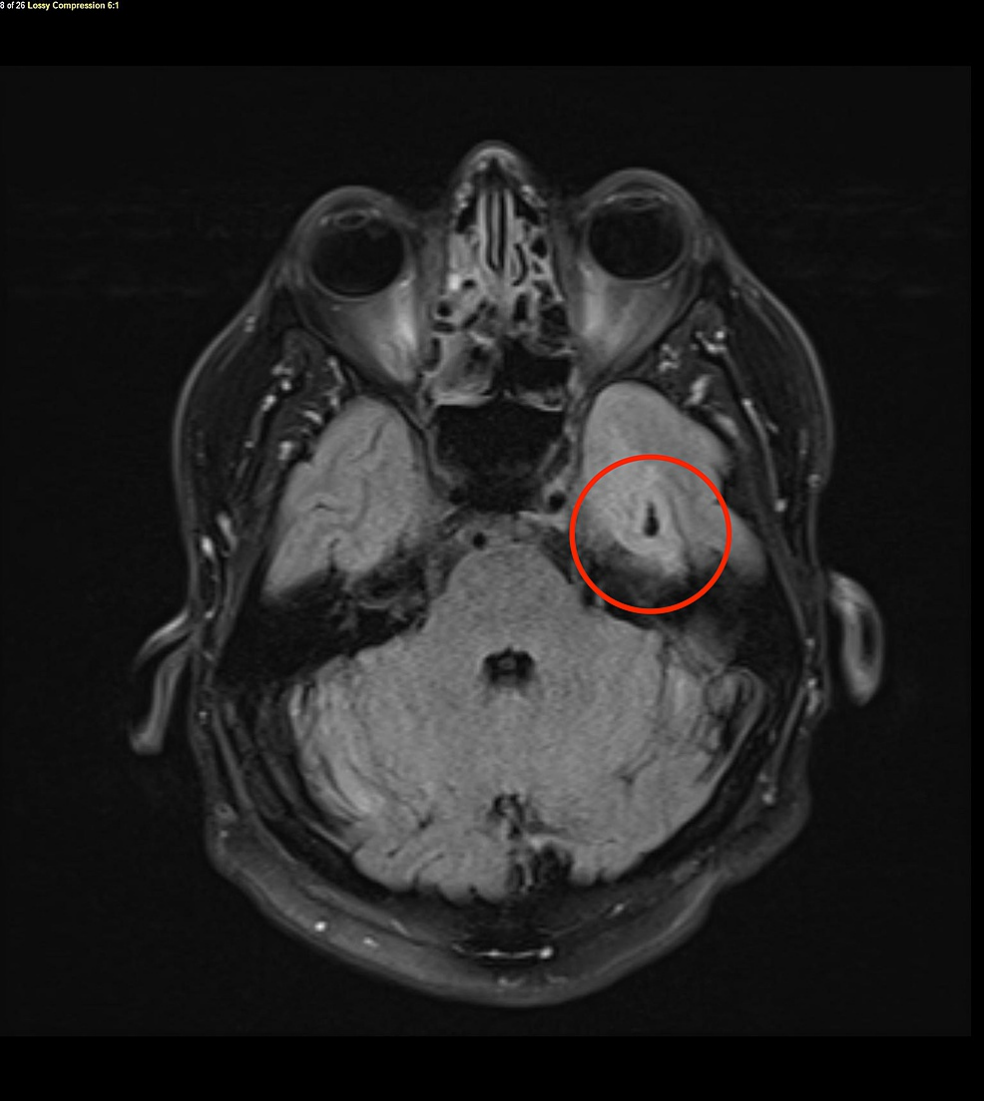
MRI of the head confirming area of left temporal encephalomalacia and gliosis (red circle).
